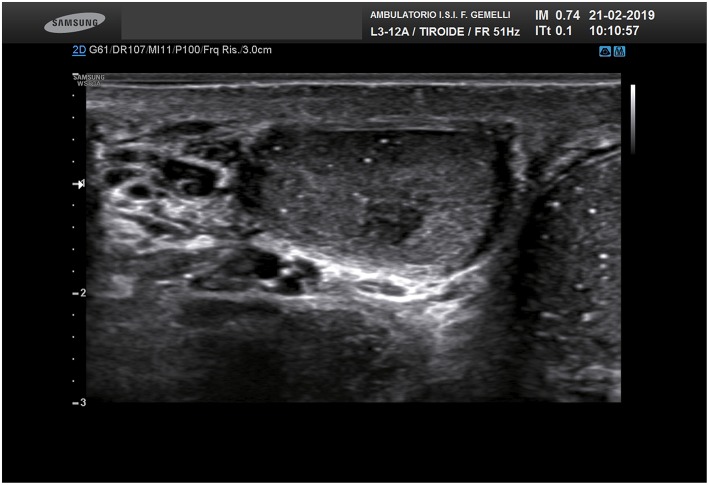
Ultrasound image of STM.
